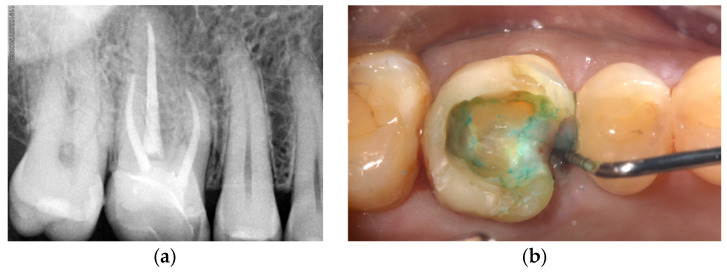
(a) Periapical radiograph of a maxillary molar with a vertical fracture, a two-wall intrabony defect, endodontic treatment and a plastic restoration. (b) Fracture line and localized increased in probing depth.
